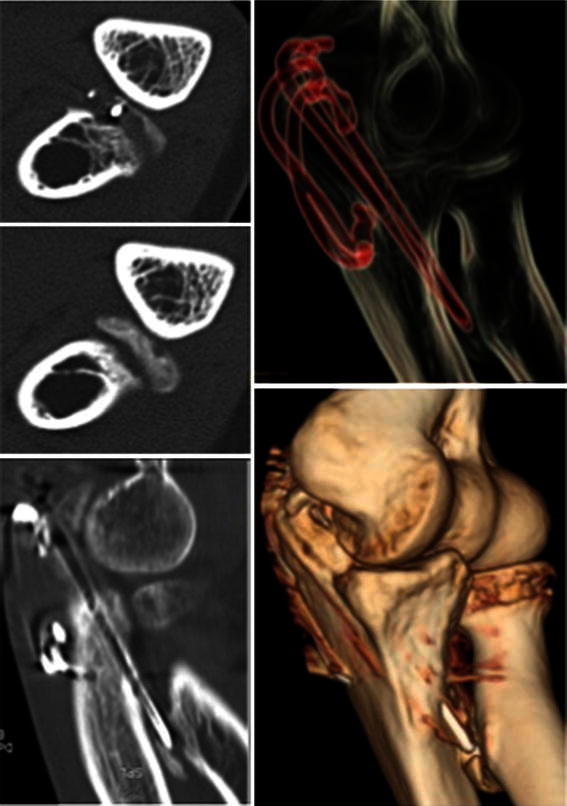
CT scan confirmed that the prominent ends of the wires were eroding the radial tuberosity with secondary heterotopic ossification between the radius and ulna. In the coronal view (*left*, *middle*). there was 1-mm gap between the heterotopic ossification and proximal ulnar shaft
